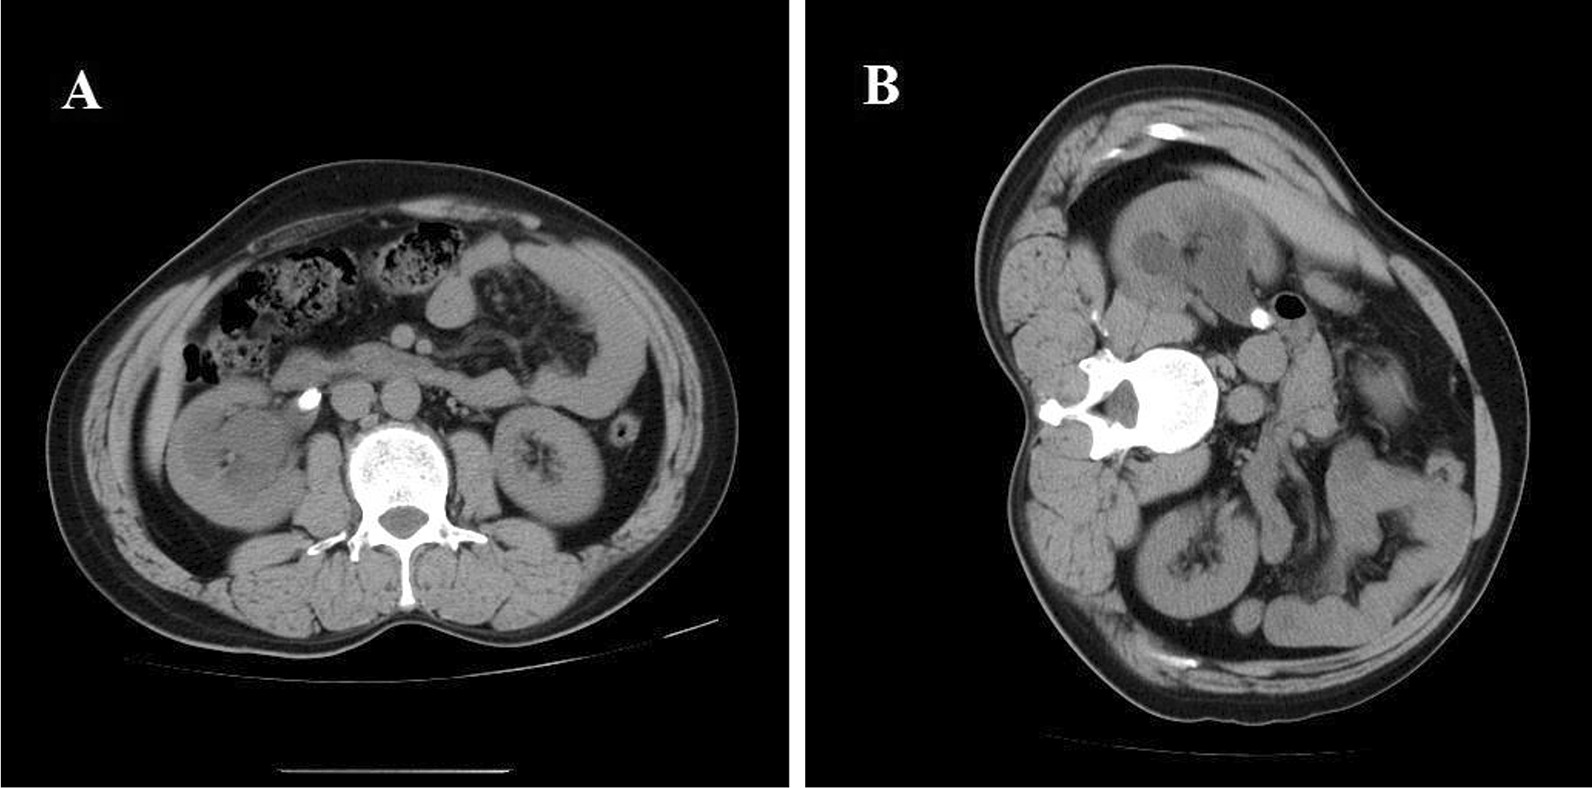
Renal pelvis shown on computed tomography (CT) images for different body positions. A In the supine position, the renal pelvis forms an angle that tends to guide the stone into the kidney if loosened during the operation. B In the lateral position, the left ureteropelvic junction moves to the lowest point. The stone tends to not migrate into the kidney and would fall back if it does
